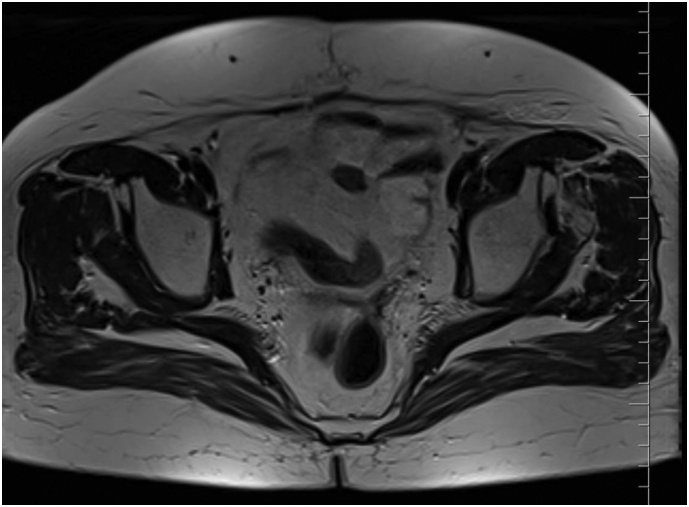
Magnetic resonance image demonstrating complete regression of lesions located at left apical part vaginal vault taken 12 months after completion of MR-guided stereotactic radiotherapy.
